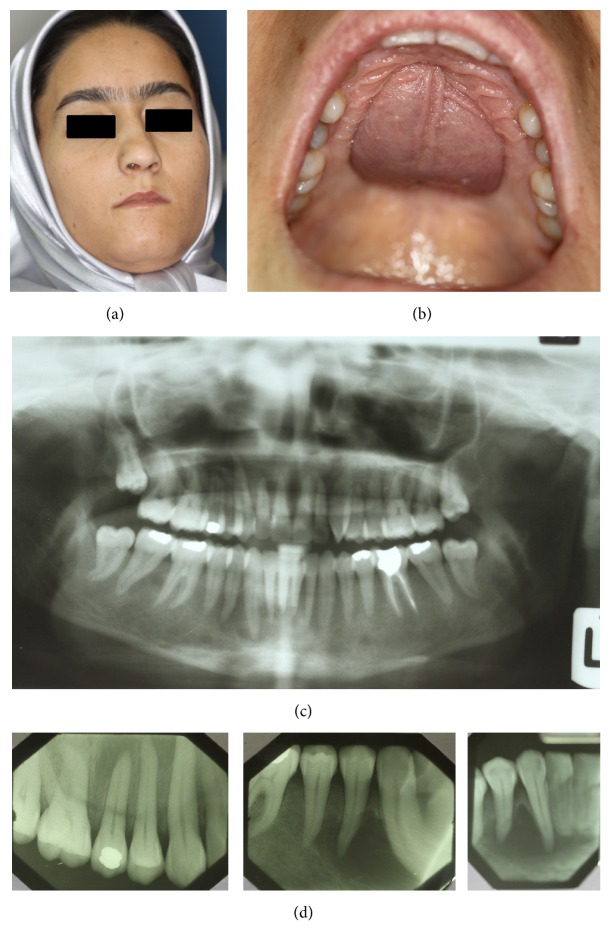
First visit: (a) extraoral frontal photography, (b) intraoral photography, and (c) panoramic and (d) periapical views.
